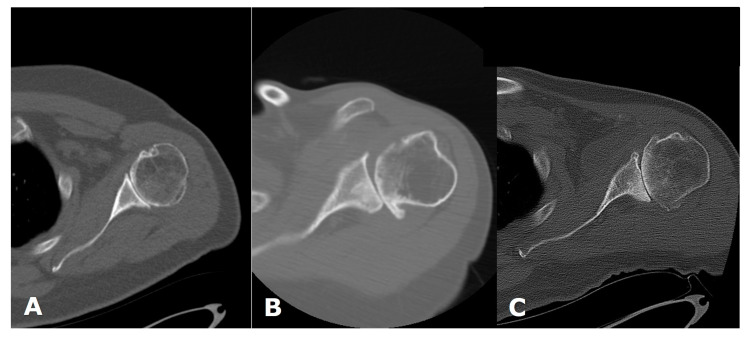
Axial computed tomography (CT) images of the shoulder in three different patients, showing different glenoid morphologies. (A). Walch A. (B). Walch B1. (C). Walch B2.
